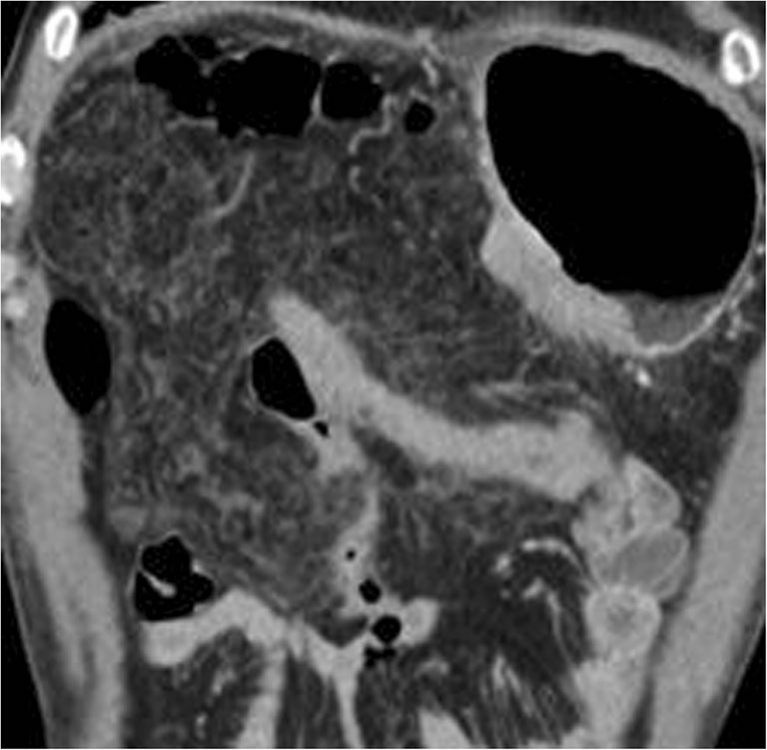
Fat stranding. Coronal CT image in the immediate postoperative period shows a diffuse stranding of the mesenteric fat tissue
